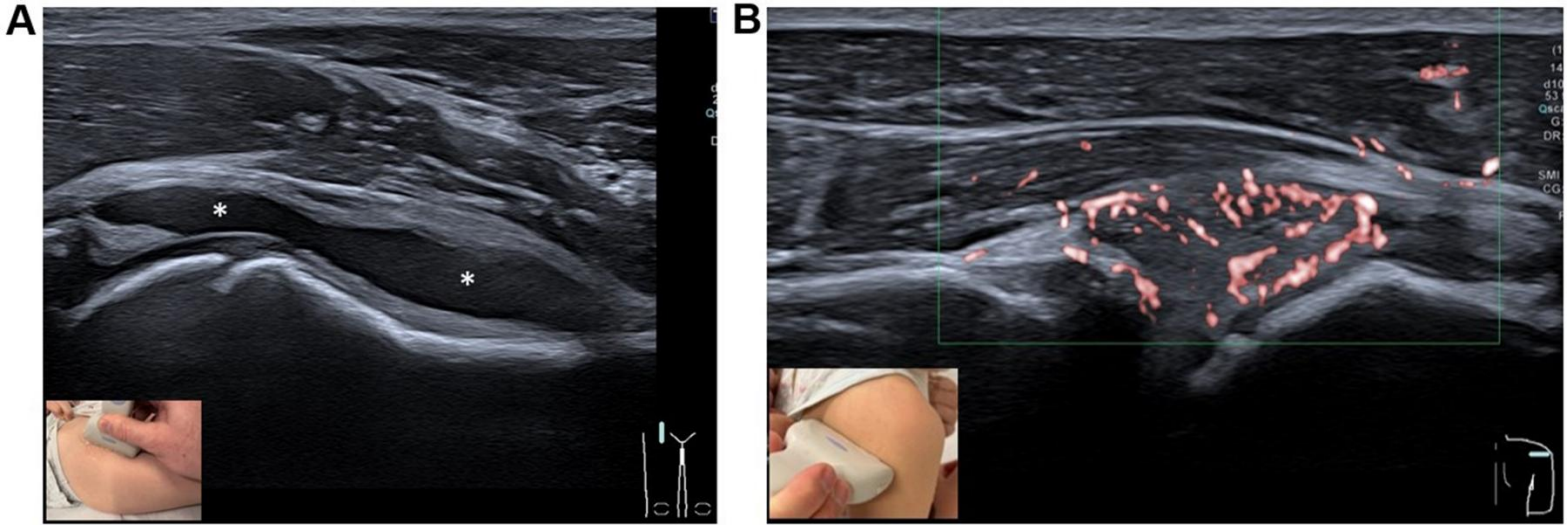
An example of B-Mode and power Doppler grade 3 in two joints with active arthritis. (A) An example of the sonography ventral longitudinal view of a hip joint with B-Mode Score Grade 3: a large significant effusion (asterisks) that leads to the development of a convex-shaped anterior capsula can be seen with extension of the effusion to the epiphysis. (B) An US image of the dorsal transverse view of the glenohumeral joint of the shoulder. Confluent intrasynovial power Doppler signals in over 30% of synovial tissue can be seen, representing an example of a joint with a power Doppler Score Grade 3 when graded using the paediatric OMERACT score
